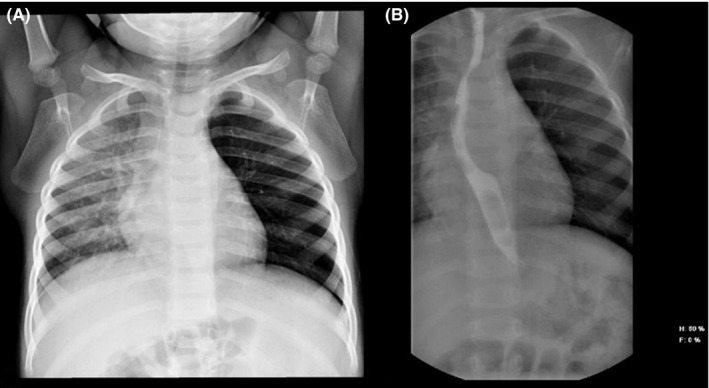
A, Chest X‐ray: diffuse abnormal hyper‐transparency of the left lung fields, with a shift of trachea and mediastinum to the right side, associated with moderate vascular congestion. B, Upper gastrointestinal tract radiography with barium shows an impression on the left side of barium filled esophagus associated with a consequent displacement on to the right side
